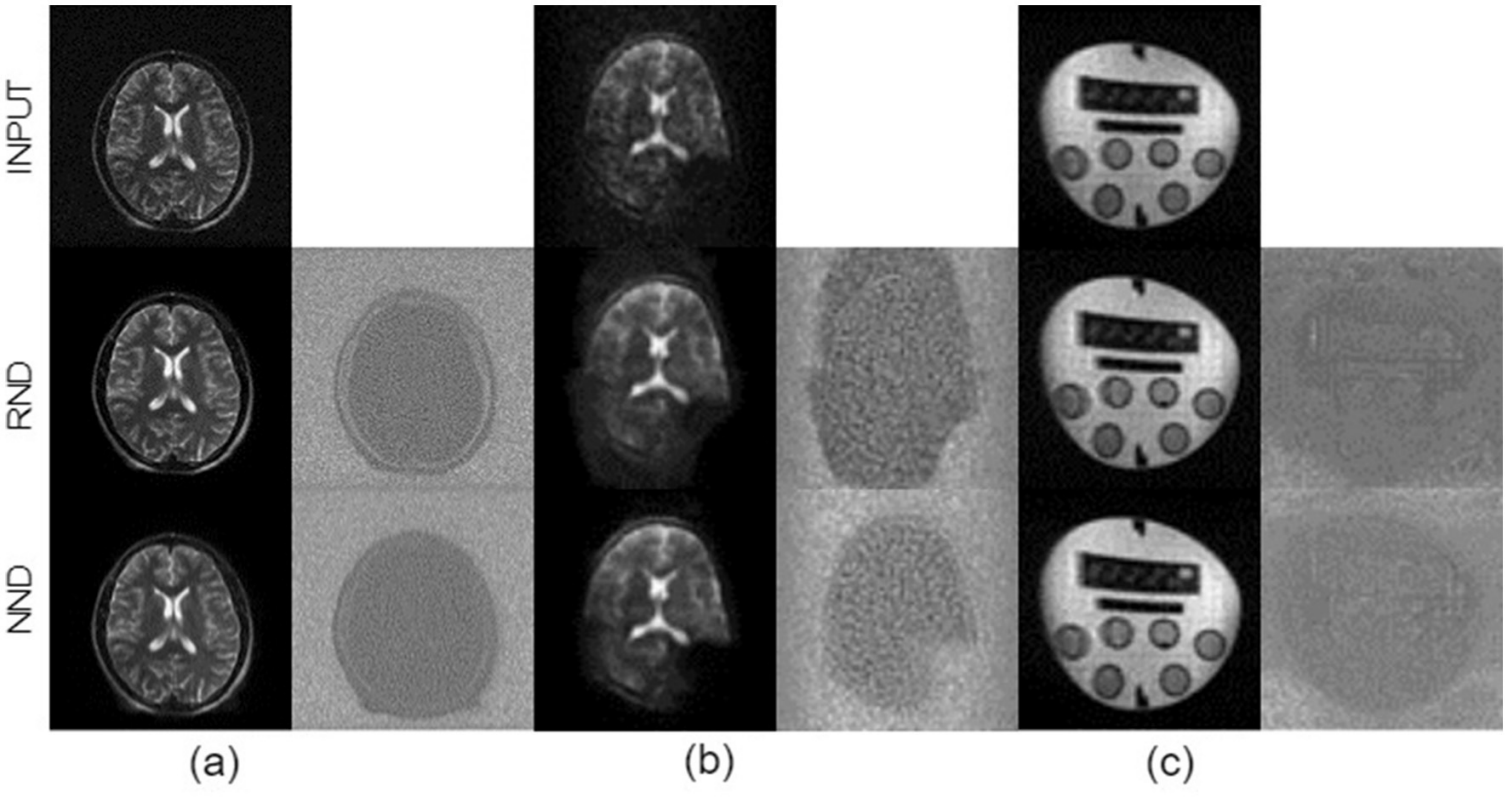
Comparison of denoising techniques across different MRI datasets for full images. The figure displays results from three datasets: (a) M4Raw, (b)
